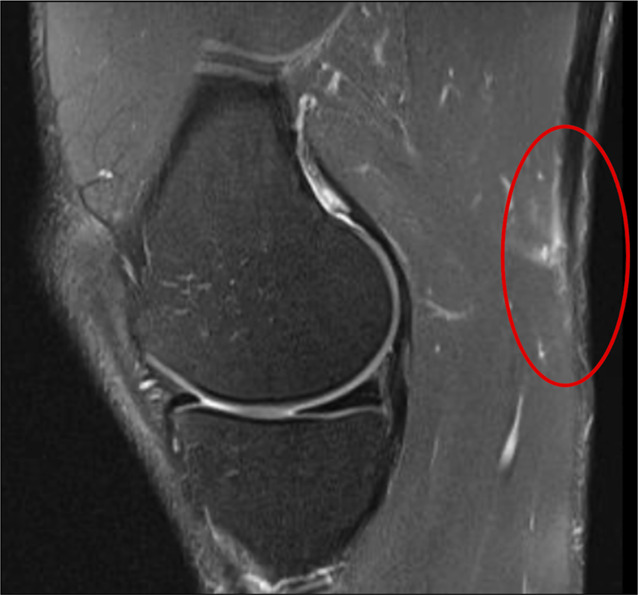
Sagittal T2 PDW MRI slice demonstrating a distal avulsion of the semitendinosus tendon with the tendon stump circled in red. The MRI was taken in the subacute phase such that there is minimal edema and/or fluid collection present.
